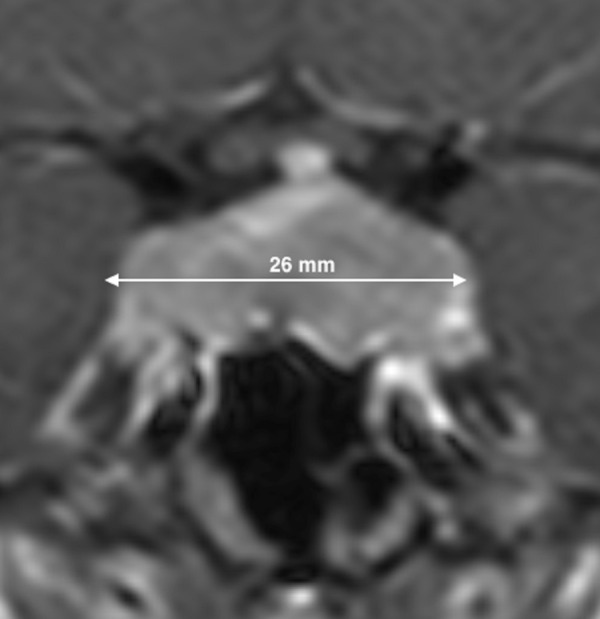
Coronal Post-Contrast MRI Sequences First recurrence despite maximal medical therapy, showing marked enlargement and invasion of both cavernous sinuses
